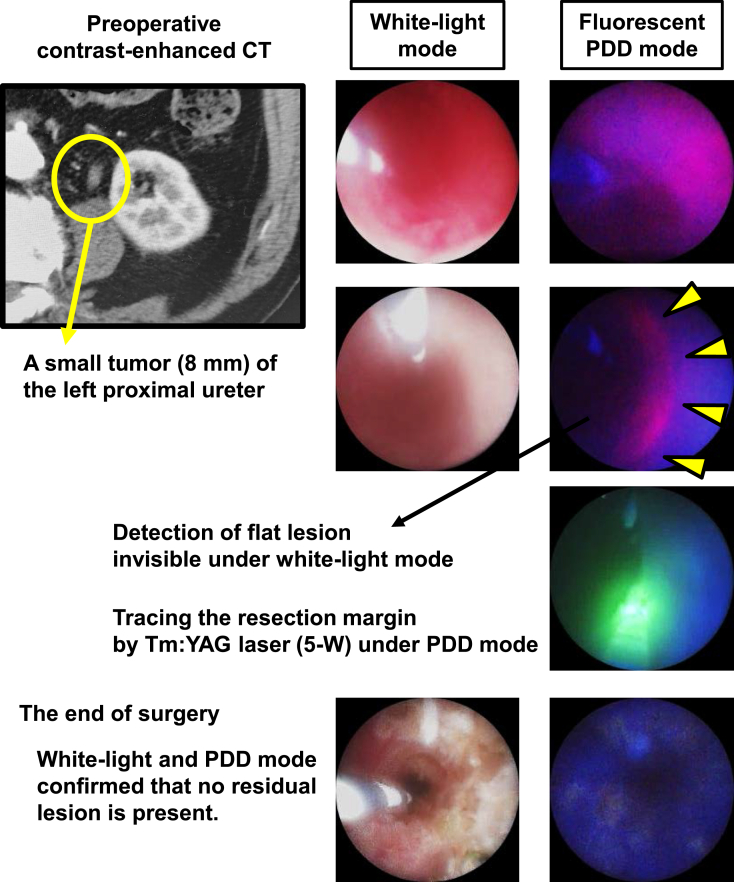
A representative case showing benefits of using photodynamic diagnosis (PDD) assistance during the ureteroscopic laser ablation (ULA). A 74-year-old man presented to a urology clinic with gross hematuria. A contrast-enhanced CT revealed an 8-mm tumor of the left proximal ureter. The ureteroscopic biopsy pathologically confirmed low-grade urothelial carcinoma. The patient was considered to be well indicated for the ALA-PDD-ULA. First, a papillary-shaped tumor is observed under white-light mode. Then, the PDD mode detects a spreading flat lesion which is invisible under white-light mode. We trace the resection margin by Tm:YAG laser (setting: 5-W) under PDD mode. At the end of surgery, white-light and PDD mode confirmed that no residual lesion is present.
